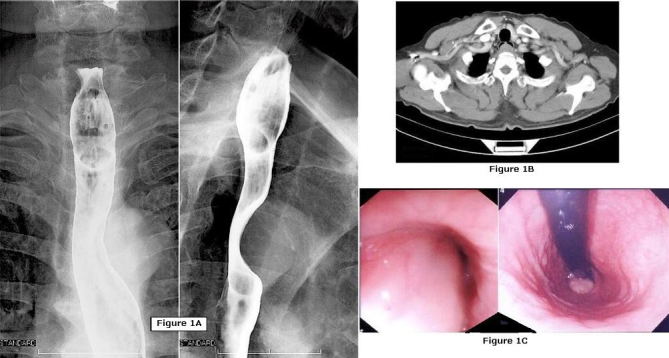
Preoperative examinations. An esophageal submucosal polypoid lesion was identified by barium esophagogram (A), CT (B), and downward (C, left) and upward (C, right) views by upper GI endoscope.
